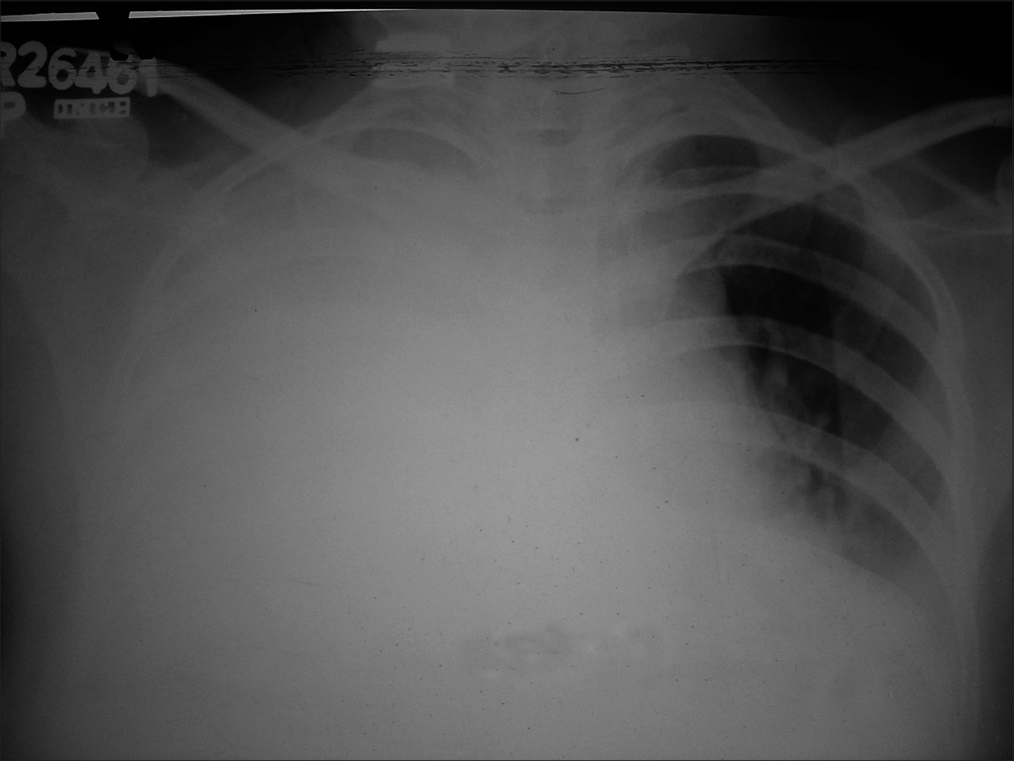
Chest radiograph showing bilateral pleural effusion and cardiomegaly
